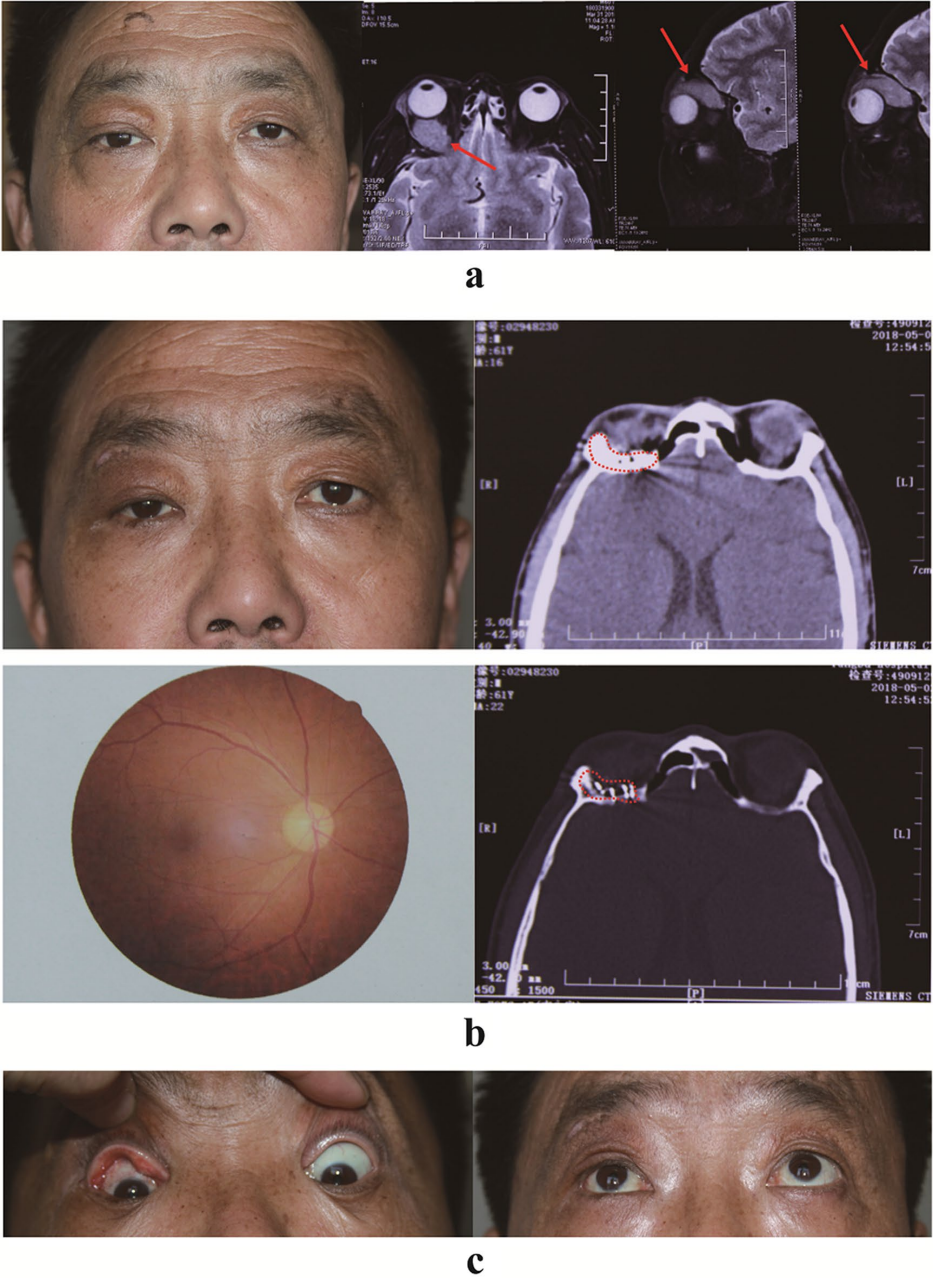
Pre- and postoperative images of patient B. a Preoperative images of patient B. The photograph of the patient's appearance shows the right eyeball protruding and fixed downward. A CT scan shows a soft tissue mass in the right superior rectus (red arrows). b Twenty-nine months after brachytherapy. The photograph of the patient's appearance shows no proptosis and no periocular radiation dermatitis. The CT scan shows that the I-125 seeds under the orbital periosteum are stable (red dashed boxes). The fundus photograph of the right eye was normal. c Eye movements (upward and downward) return to normal
